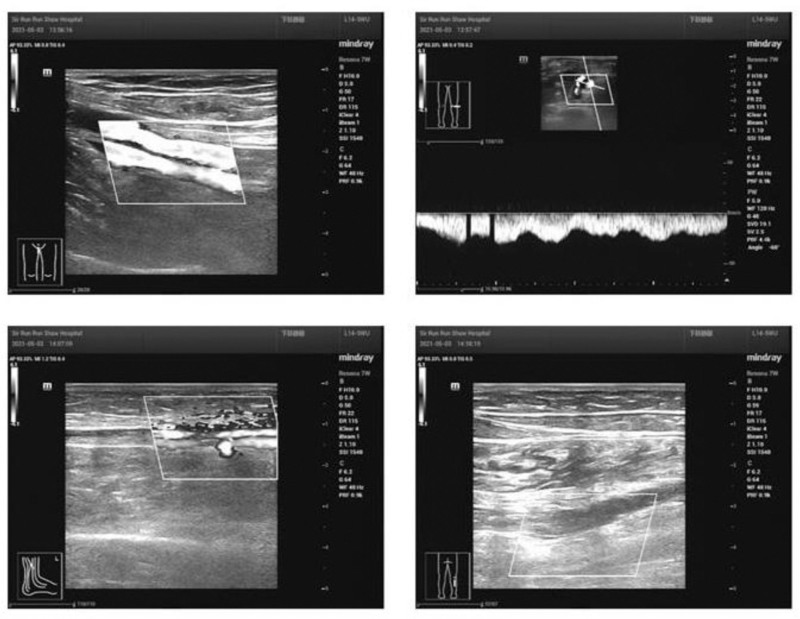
Venous duplex ultrasonography confirming lower extremity venous intermuscular thrombosis.
